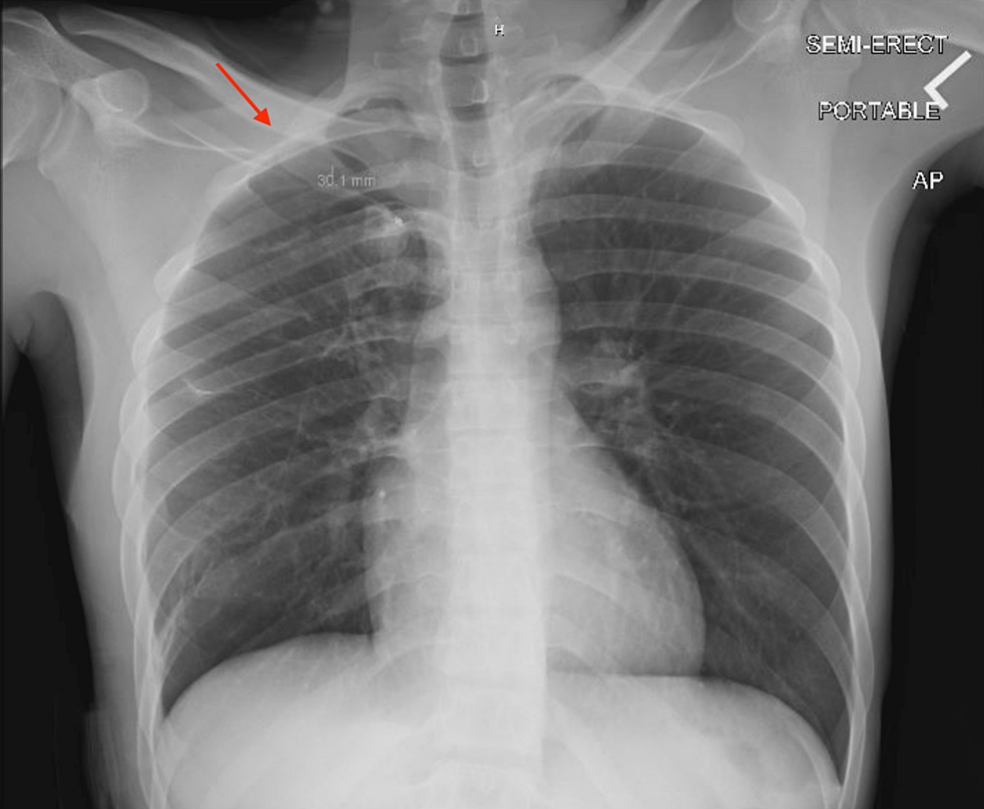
Chest X-ray showing stable small right apical pneumothorax
